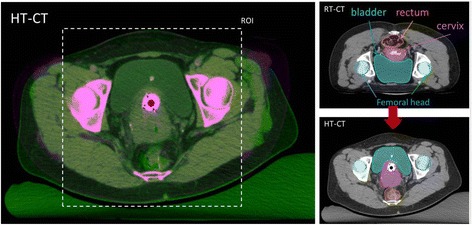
Image registration/matching. Radiotherapy and hyperthermia CT scans are rigidly matched by visual assessment of the bony anatomy, followed by deformable registration using intensity-based deformable image registration software of Velocity Medical Solutions (Varian Medical systems, Palo Alto). Left: overlay showing the excellent match of the RT-CT onto the HT-CT in the Region of Interest (ROI) indicated with the dotted rectangle. Right: Outlines of rectum, cervix, bladder and part of the bony anatomy are shown in the RT-CT and the HT-CT
